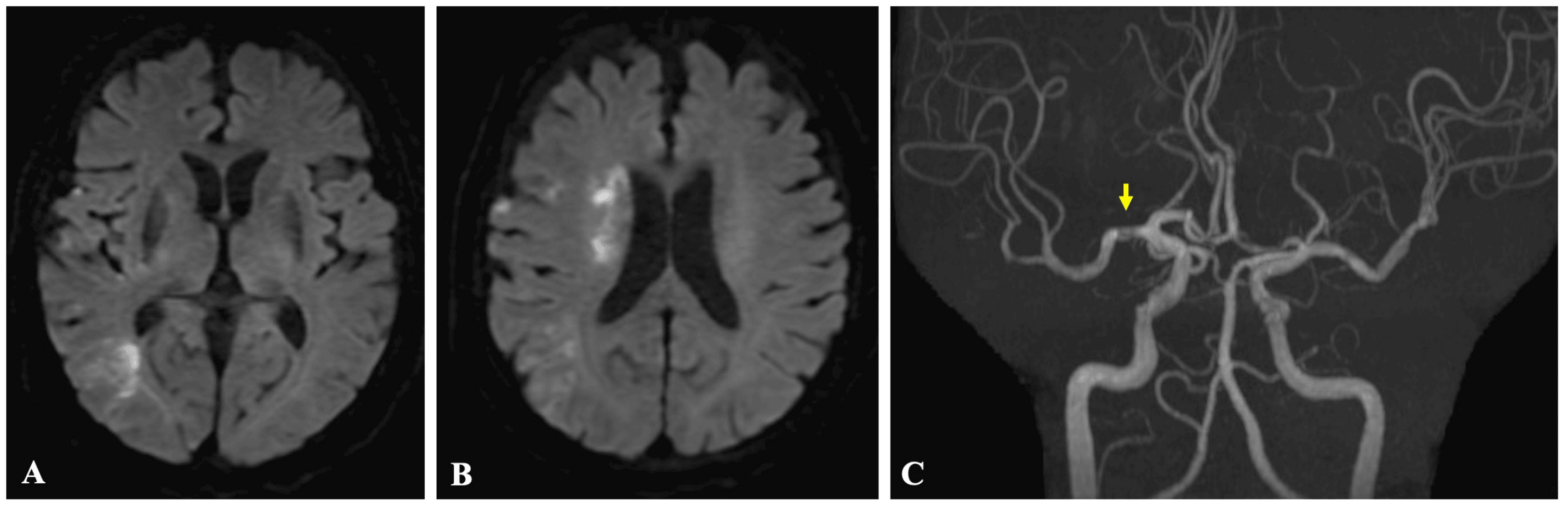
(A and B) Brain magnetic resonance imaging and (C) angiography at discharge Axial diffusion-weighted images show no new ischemic or hemorrhagic lesions and stable findings in the right MCA territory (A and B). Magnetic resonance angiography demonstrates preserved right M1 patency without evidence of dissection or restenosis, indicating maintained recanalization (C, arrow)
